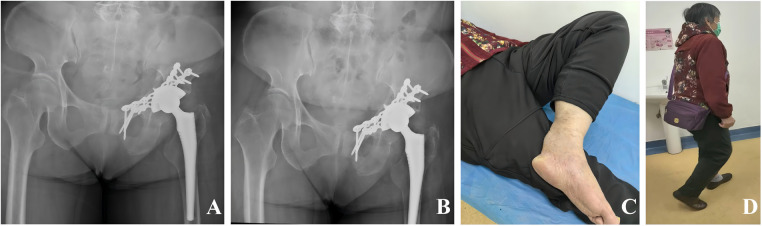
Postoperative follow-up images. (A) postoperative anteroposterior x-ray images of the pelvis at 1 month postoperatively. (B) postoperative anteroposterior x-ray images of the pelvis at 6 months postoperatively. (C) Image of hip flexion at 6 months after surgery. (D) Image of standing and walking at 6 months after surgery.
